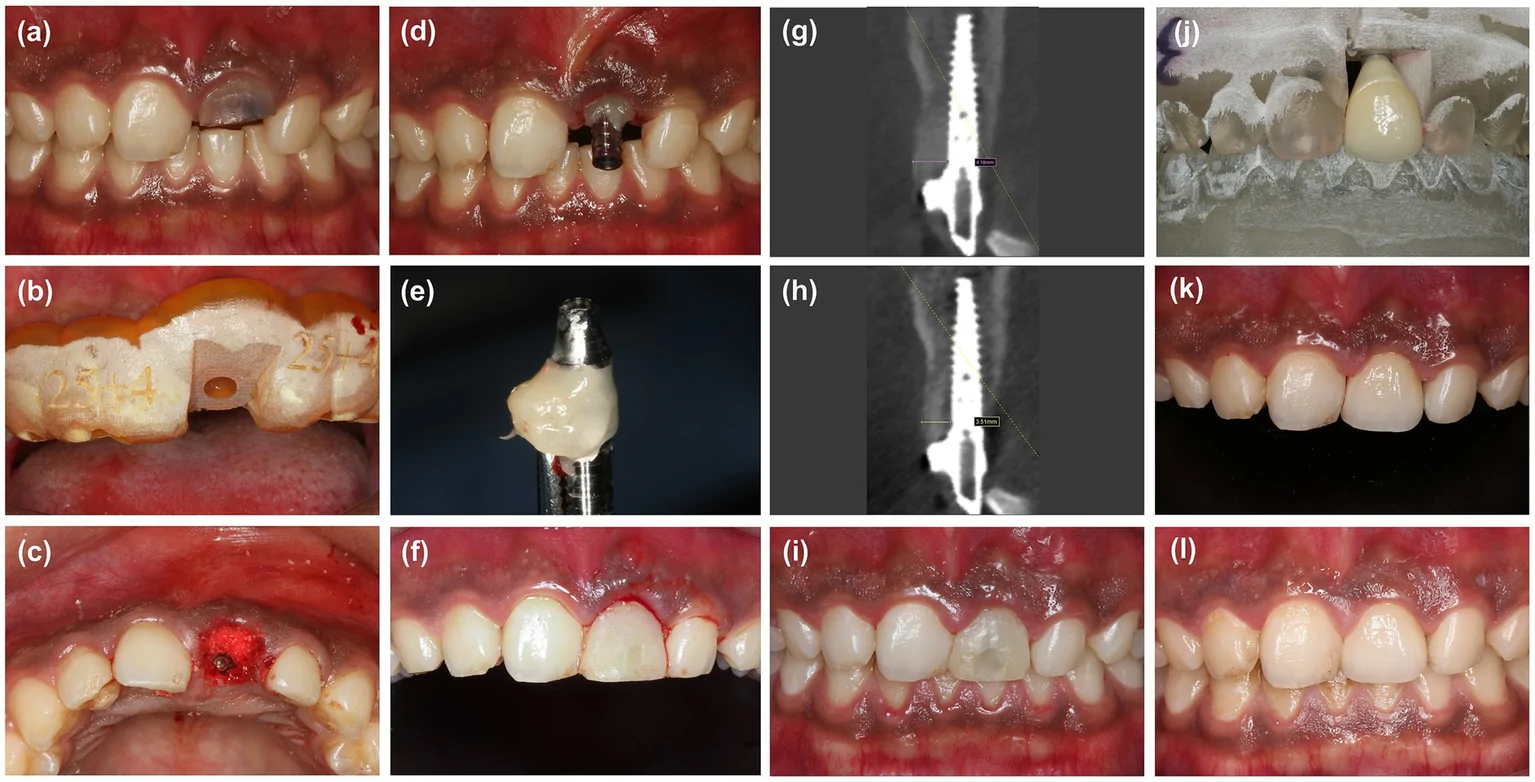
(a) Intraoral view before the surgery. (b,c) Implant placement guided by a surgical template. (d–f) Immediate provisionalization. (g) CBCT image immediate post-operation. (h) CBCT image at 3 months. (i) Intraoral view at 3 months. (j,k) Delivery of the final prosthesis. (l) Intraoral view at 6 months.
